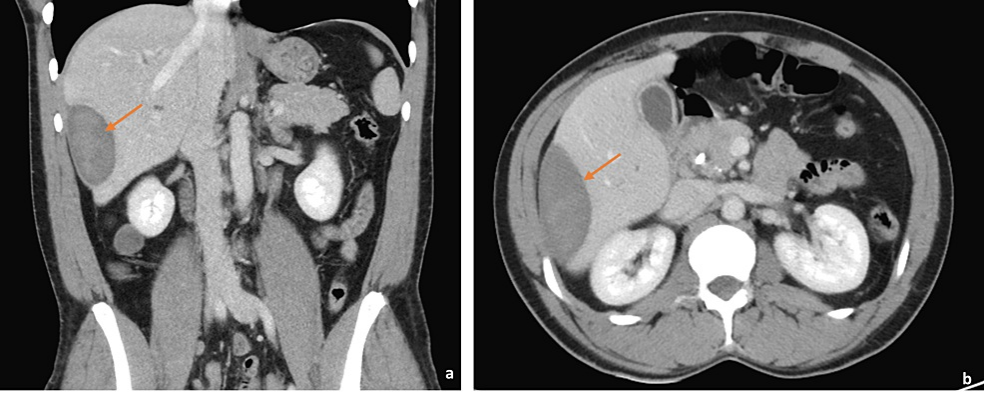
A triple-phase computed tomography scan of the abdomen and pelvis demonstrating a right-sided subcapsular hematoma in segment 6 of the liver within the (a) coronal and (b) transverse planes (arrow).
